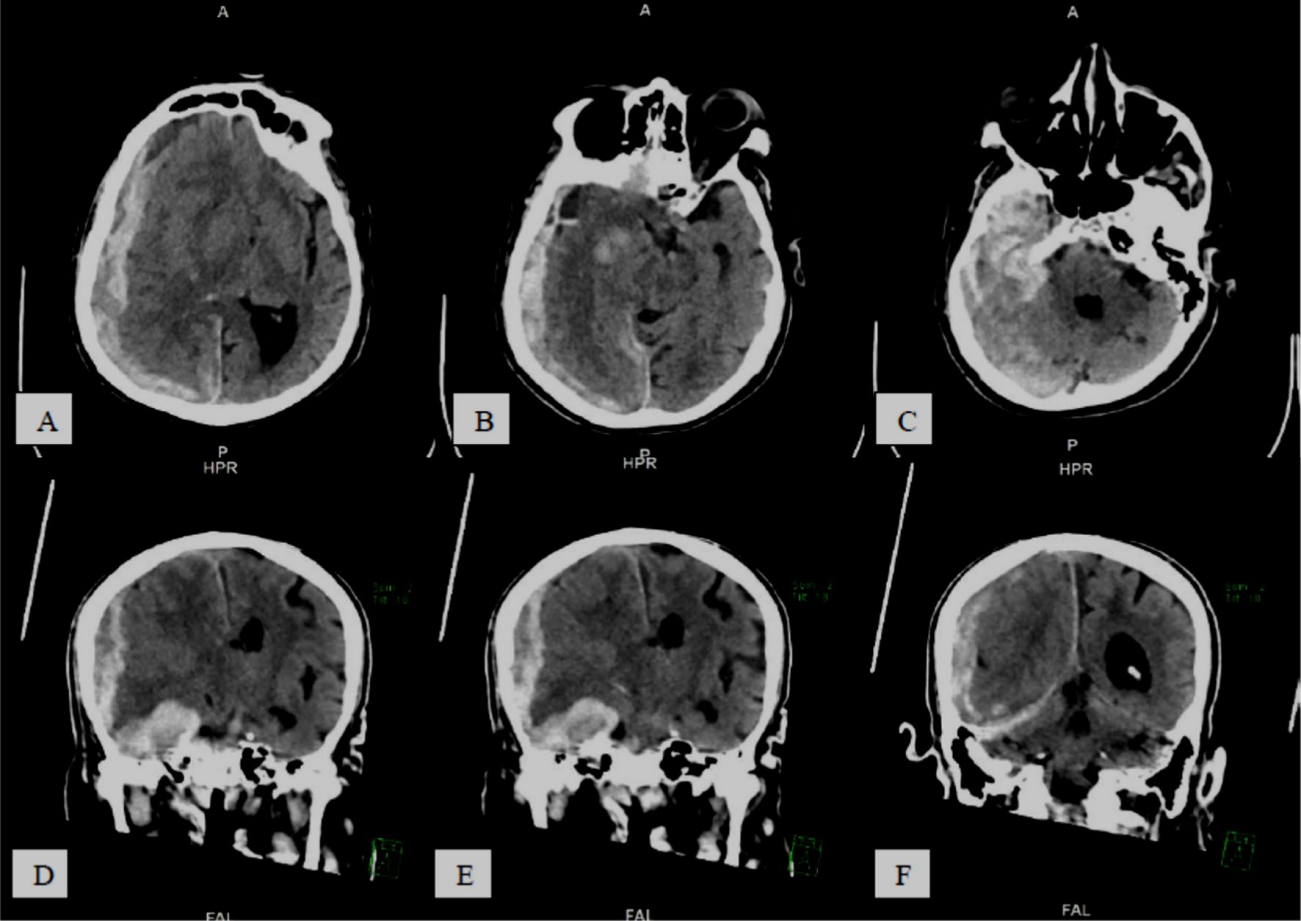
88-year-old COVID-19 male patient with sudden onset of left sided weakness and syncopal attack, under umbrella of anticoagulant, A–C and D–F serial axial and coronal CT images, respectively, show right fronto-temporo-parital extra-axial acute on top of chronic subdural hematoma with mid line shift, sub-falcine and uncal herniations
